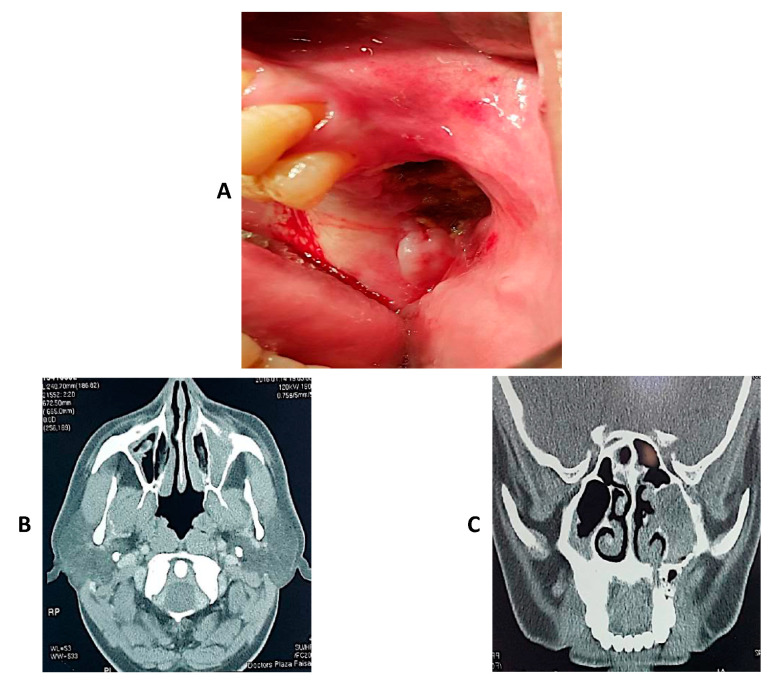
Picture A, showing exposed necrotic bone in the left posterior maxilla (A). The axial (B) and coronal (C) slices of contrast enhanced CT scan showing bone destruction, sequestrum formation, sinus opacification, and involvement of the nasal turbinates.
